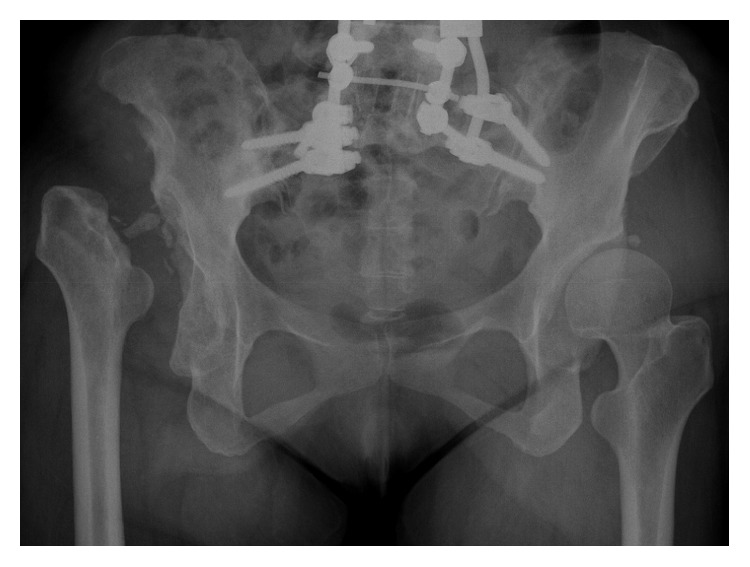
Case 1 preoperative hip X-ray. Right Charcot arthropathy of the hip shows typical radiographic changes.
